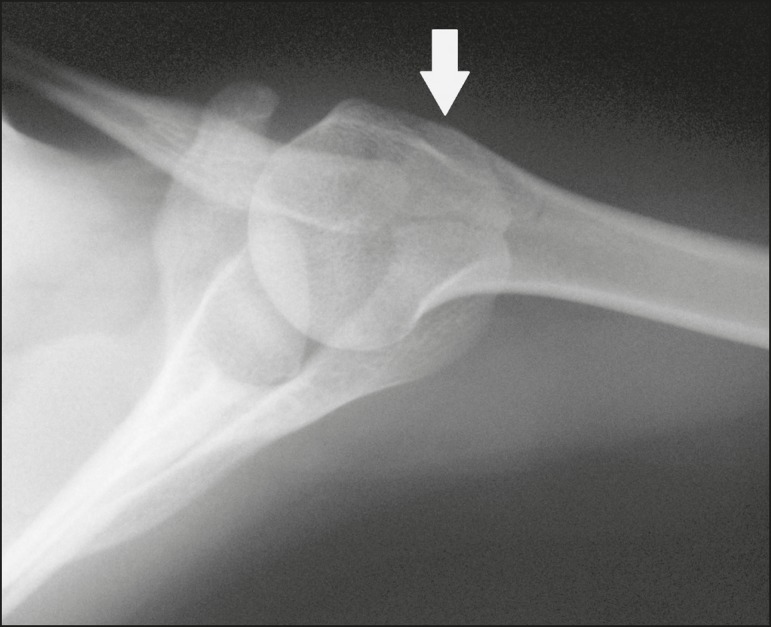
Axillary X-ray view showing a triangular ossicle distal to the acromion
